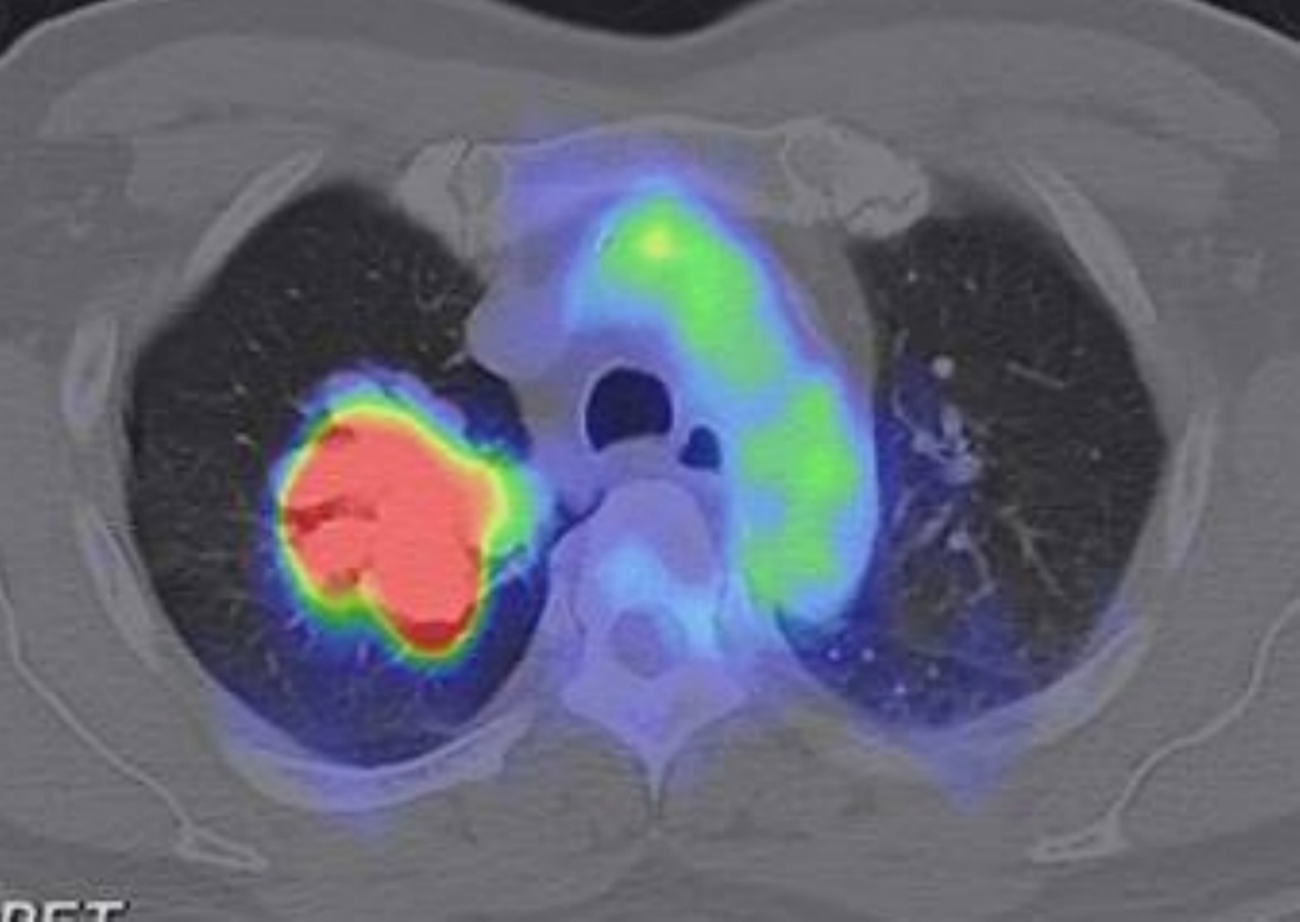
Imaging findings. PET‐CT showed an FDG‐avid mass in the right upper lobe with a maximum standardised uptake value of 12.8. CT, computed tomography; PET, positron emission tomography; FDG, ^18^F‐fluorodeoxyglucose.
